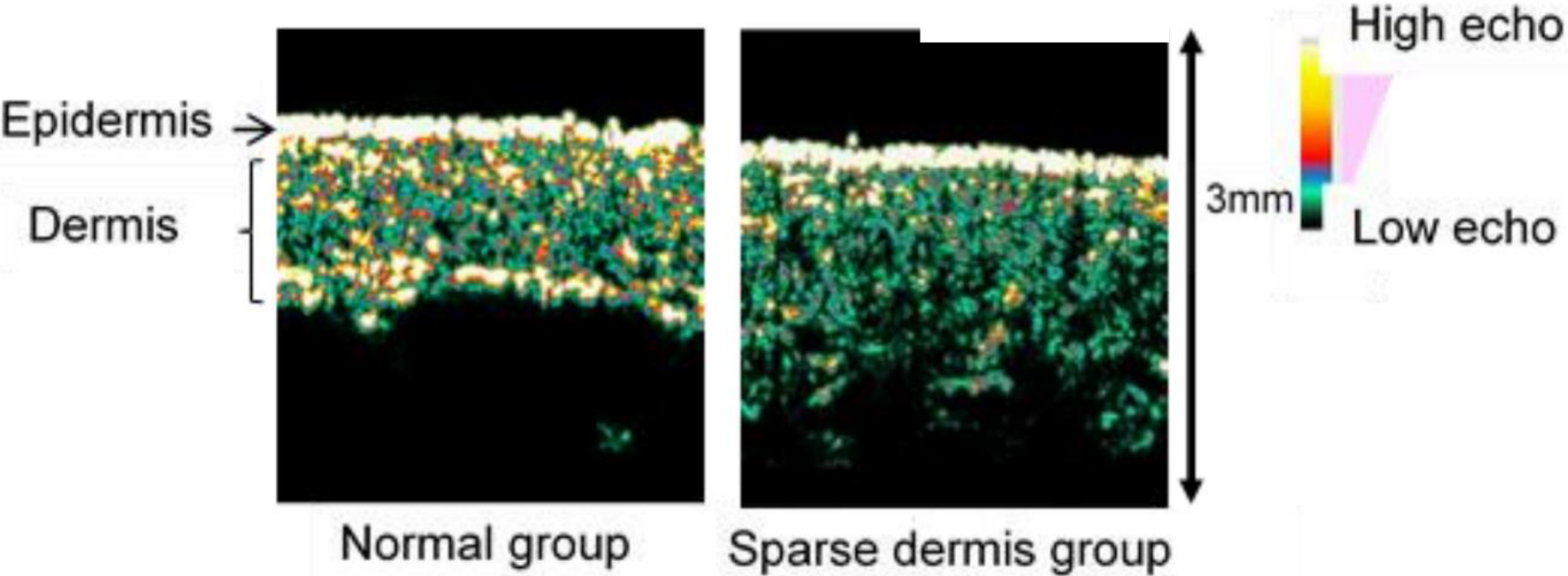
Ultrasound images. We classified ultrasound images into two types. Left panel: normal group where the hyperechoic area is present in the entire dermis; and Right panel: sparse dermis group where the hyperechoic area is absent in part of or the whole dermis.
